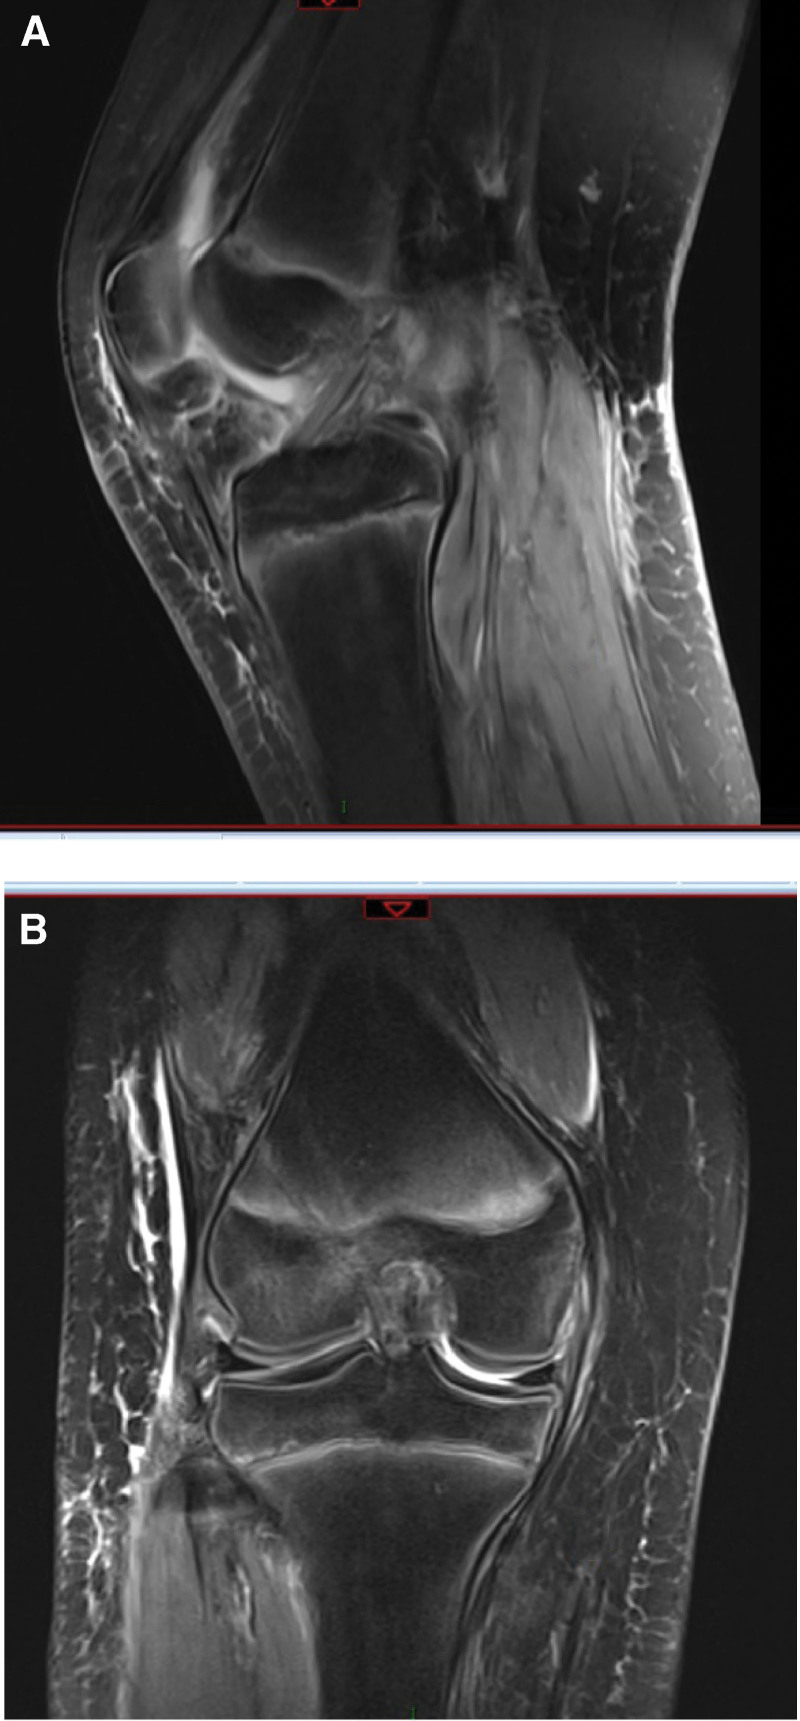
(A) An MRI scan image of the Sagittal side revealed good morphology of the ACL following the definitive fixation; (B) An MRI scan image of the Coronal side showed good morphology after the definitive fixation.
